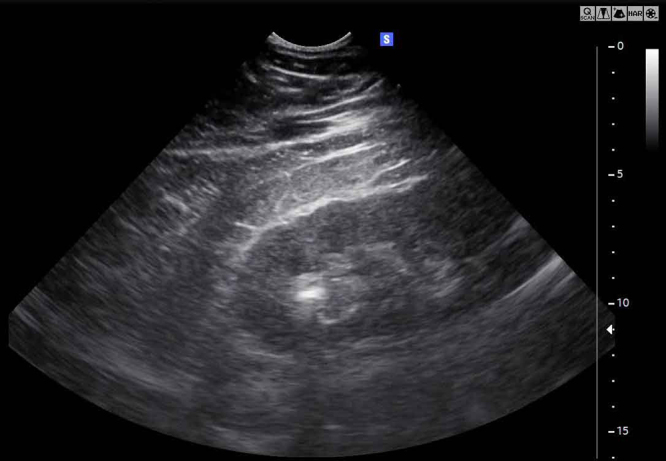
Interfaces of various tissue layers in front of a kidney with a stone are depicted in an ultrasound echo image. Ultrasound and shock waves both are acoustic waves and are partly transmitted and partly reflected according to the reflection coefficient *R* for the present impedance difference. For the tissue layers in front of the kidney, transmission of the ultrasound waves dominates due to similar *Z*-values (*Z*_2_ ≈ *Z*_1_), for the stone, reflection of ultrasound reflection dominates due to significantly different *Z*-values (*Z*_2_ ≠ *Z*_1_). The lower part of the image shows a bright stone reflex, which is followed by a dark shadow, indicating that almost no ultrasound had passed the stone.
